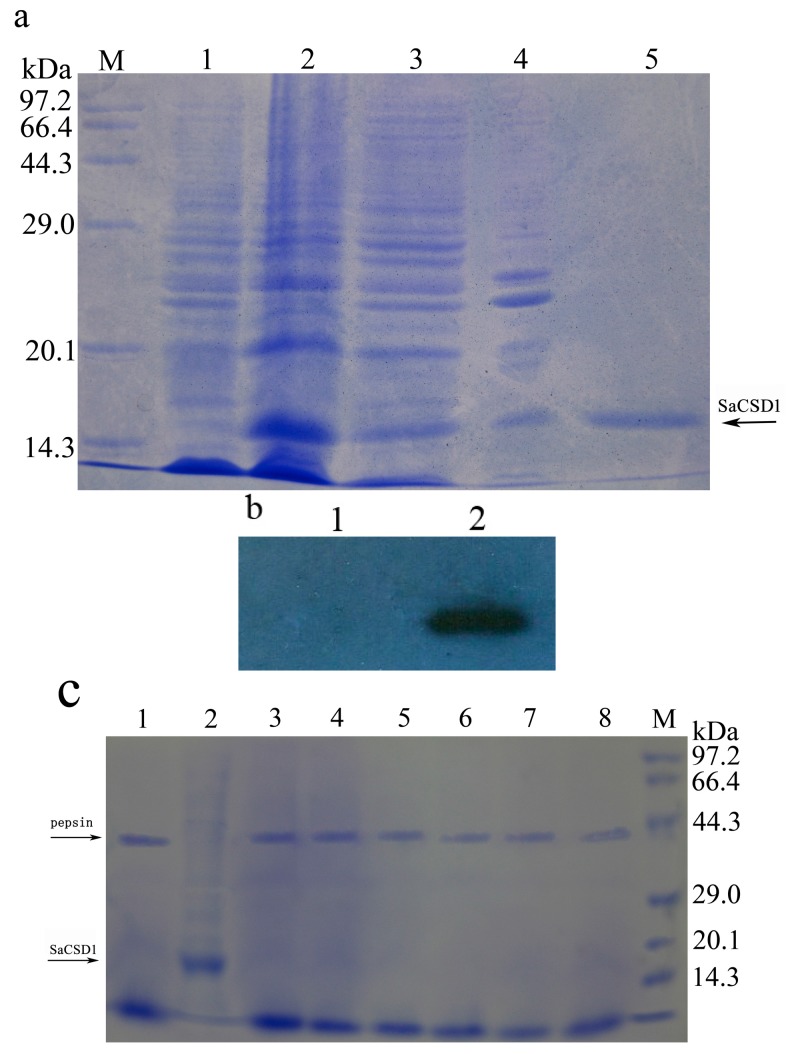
Expression, purification, Western Blot and pepsin digestion analysis of recombinant SaCSD1 protein. (a) Electrophoretogram of SaCSD1 protein. Lane M, protein marker; lane 1, uninduced *E. coli* lysate; lane 2, IPTG-induced *E. coli* lysate; lane 3, supernatant of the lysis after ultrasonication; lane 4, pellets of the lysis after ultrasonication; lane 5, the purified SaCSD1; (b) Western Blot detection of fusion protein with anti-His mAb. Lane 1, uninduced *E. coli* lysate; lane 2, the purified SaCSD1; (c) *In vitro* standardized pepsin digestion assay of SaCSD1 protein. Lane 1, the reaction without SaCSD1; lane 2, the reaction without pepsin; lane 3–8, the reaction in different times (0.5, 2, 5, 10, 20, 30 min); M, protein marker.
